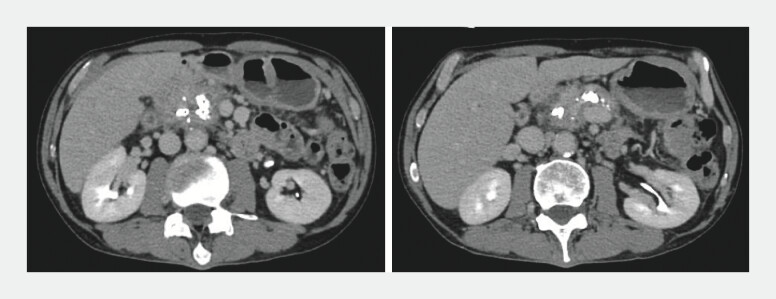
Contrast-enhanced computed tomography revealed the dilatation of the main pancreatic duct and multiple pancreatic stones around the endoscopic pancreatic stenting.
